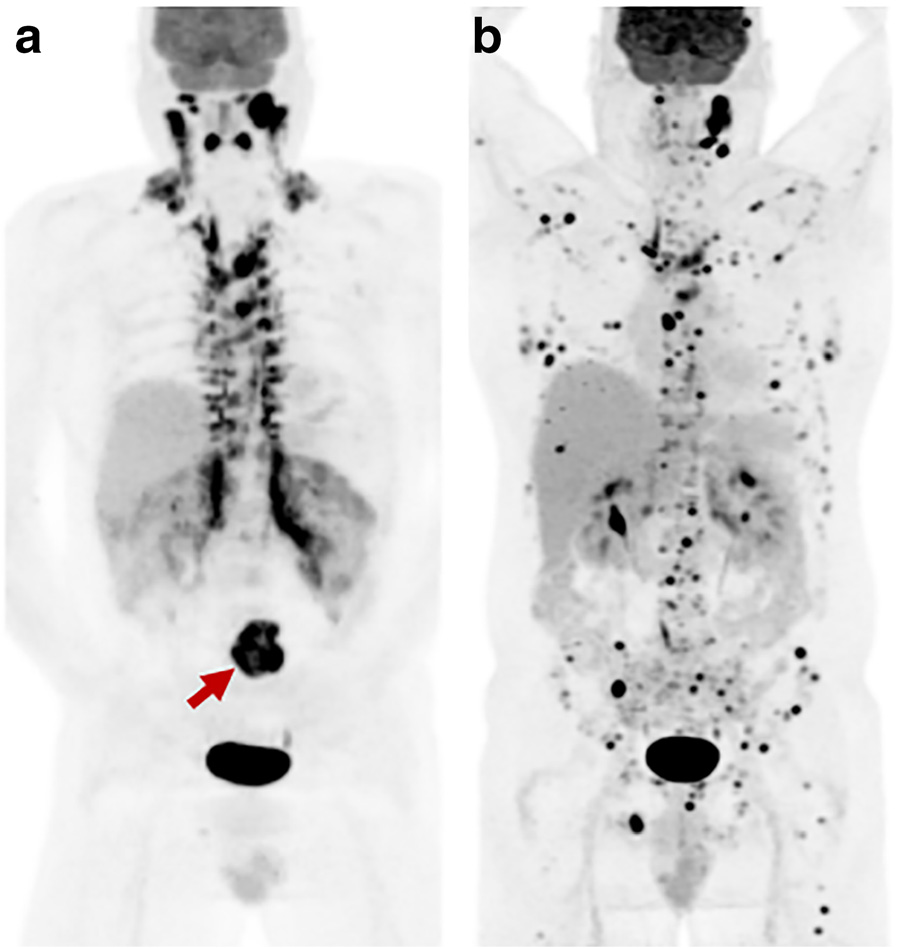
The same patient from Fig. 7 with baseline F-18 FDG (a) shown prior to commencement of alpha/beta-blockade. Following excision of the primary site (*red arrow*) there was marked progression of metastatic disease on FDG (b) with concordant biochemical progression. Despite rising normetadrenaline levels (>100,000pmol/L compared to 18,000pmol/L at baseline) brown fat activation is not visualised following commencement of alpha/beta-blockade
